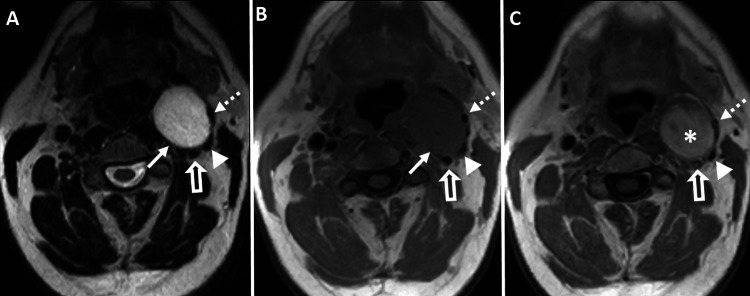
MRI of the soft tissue neck Axial images: (A) T2-weighted (repetition time msec/echo time msec, 11636/126, 5-mm section thickness), (B) unenhanced T1-weighted (repetition time msec/echo time msec, 634/16, 5-mm section thickness), and (C) contrast-enhanced T1-weighted (repetition time msec/echo time msec, 634/16, 5-mm section thickness) after intravenous injection of 16 mL of gadoteridol (ProHance; Bracco). The MRI images demonstrate a well-circumscribed, oval-shaped, homogeneously T2 hyperintense, and T1 hypointense lesion in the left carotid space (solid white arrows in images A and B). The mass causes left postero-lateral displacement of the left internal carotid artery (open white arrows in images A-C), left external carotid artery (dashed white arrowheads in images A-C), and internal jugular vein (solid white arrowheads in images A-C). The lesion exhibits near-complete contrast enhancement (white asterisks in image C) with minimal splaying of the internal and external carotid arteries.
